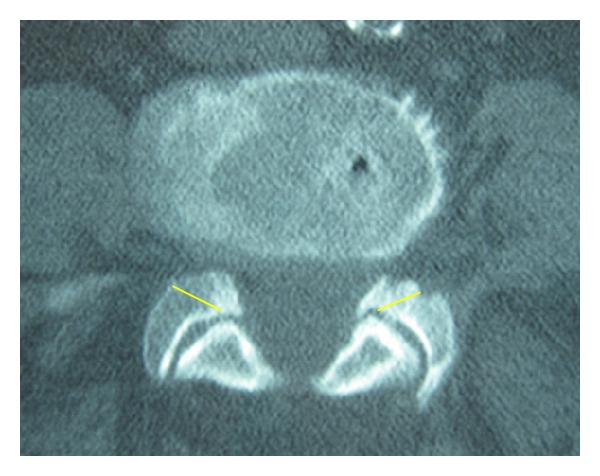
Axial CT scan image demonstrating foraminal stenosis and the planned bone resection.
